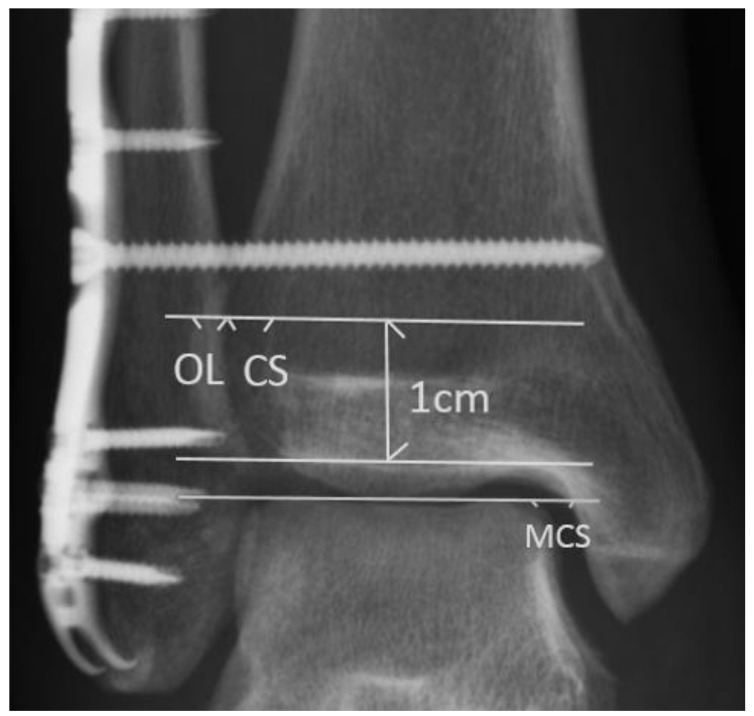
Radiographic evaluation in the study. The tibiofibular clear space (CS) was defined as the distance between the lateral border of the posterior tibial malleolus and the medial aspect of the fibula, measured 1 cm proximal to the tibial plafond. The medial clear space (MCS) was defined as the distance from the lateral border of the medial malleolus to the medial border of the talus at the level of the talar dome. The tibiofibular overlap (OL) was measured from the lateral border of the anterior tibial prominence to the medial fibula 1 cm proximal to the tibial plafond.
